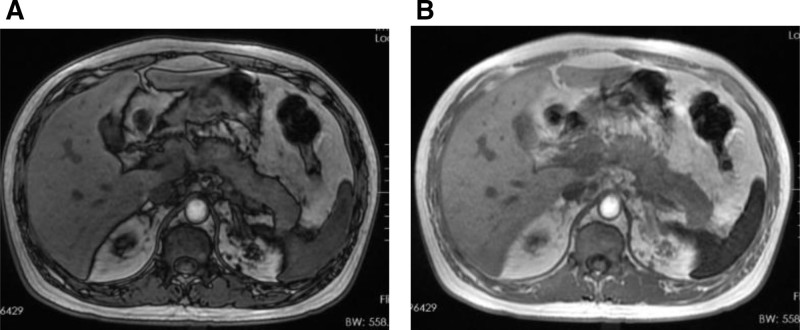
Patient’s pancreatic MRI. (A, B) The body and tail of the pancreas are mildly enlarged, exhibiting slightly prolonged T1 and T2 signals. On diffusion-weighted imaging (DWI), it shows slightly elevated signals. The enhancement is slightly nonuniform on the contrast-enhanced scan. DWI = diffusion-weighted imaging, MRI = magnetic resonance imaging.
